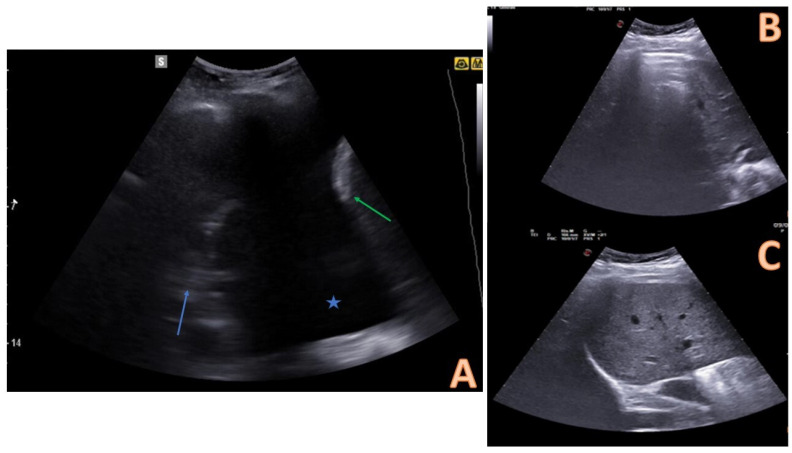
Comparison between pleural effusion (A) and healthy lung on ultrasound (B,C). In (A), the pleural effusion is visible below the chest wall and ribs as an anechoic space (blue star). The effusion is delimited inferiorly by a hyperechoic formation that represents the diaphragm (green arrow), and superiorly by an echogenic structure that identifies the atelectatic lung parenchyma (blue arrow). (B,C) represent the appearance of the healthy lung base on ultrasound: an air interface with A (horizontal) lines is evident. This artefactual image covers the abdominal organ in inspirium (B) and uncovers it in expirium (B). This sign is called a “curtain sign.” When a pleural effusion is present, the curtain sign cannot be observed.
